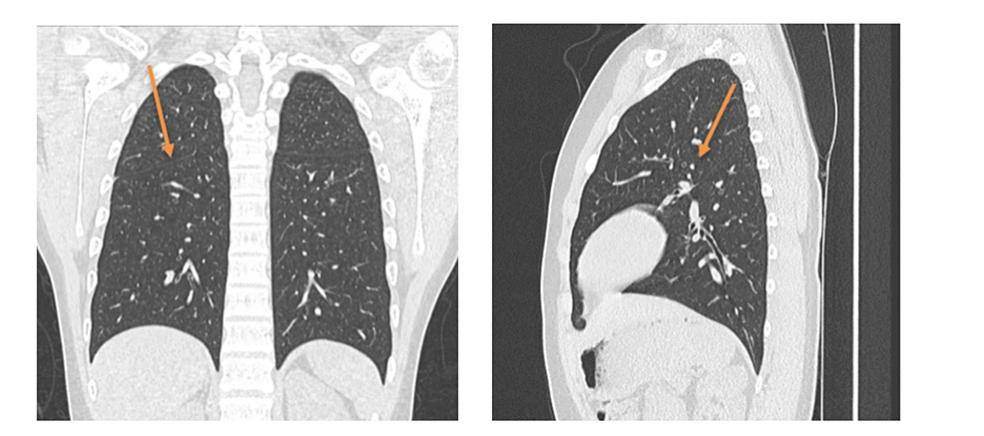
High-resolution computed tomography (HRCT) coronal and sagittal views done three months post-discharge from the hospital show full recovery of the lungs
